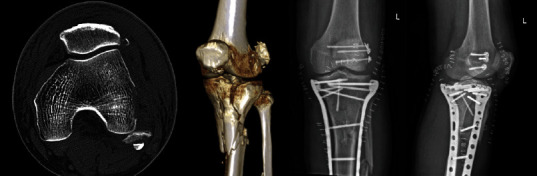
A case of extending type 2 (EX2) avulsion of lateral femoral condyle fixed by lag screws: (a) preoperative axial view CT imaging; (b) preoperative three-dimensional CT imaging; (c, d) postoperative A-P view X-ray.
